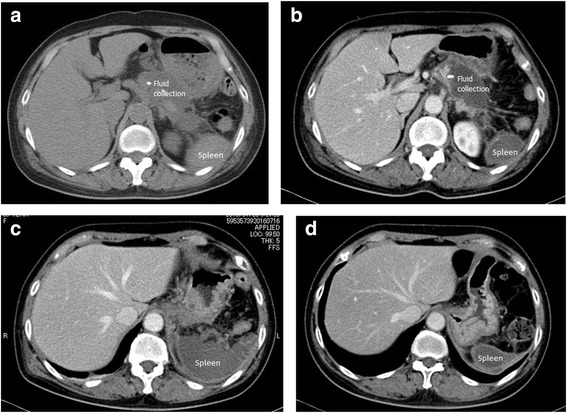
CT findings after surgery. a Fluid collection is evident around stump of the pancreas on postoperative day9. b, c Contrast-enhanced CT on postoperative day 15 revealed that the splenic artery and vein have been obliterated and complete splenic infarction is apparent. d Contrast-enhanced CT on 3 months post-operatively showed that the spleen has become atrophic and organized
